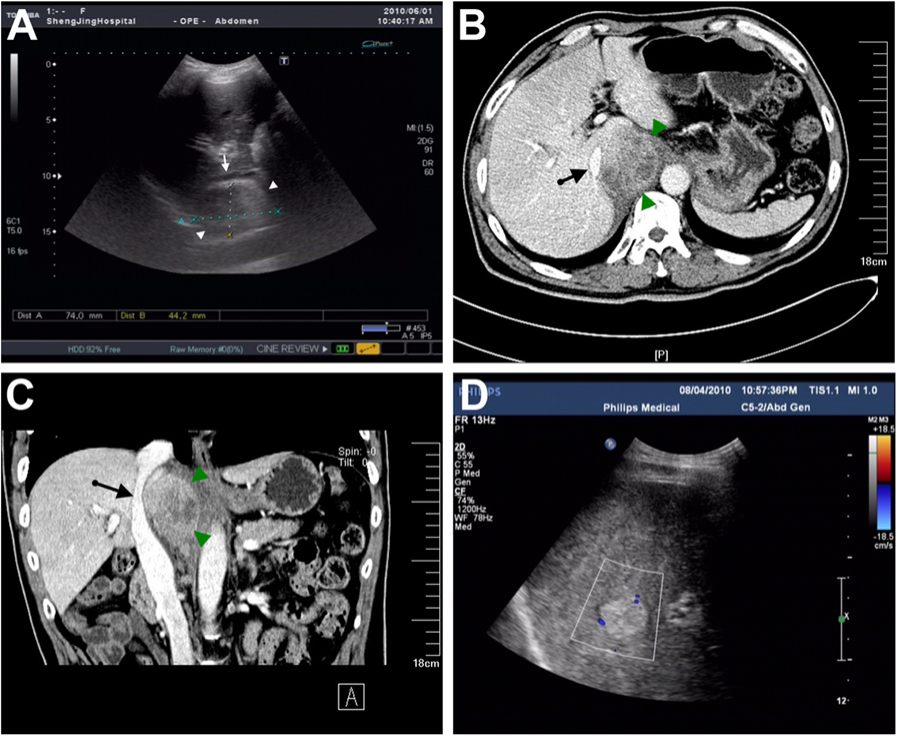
Diagnostic imaging of the retroperitoneal paraganglioma. (A) Abdominal ultrasonography showed a retrocaval mass (indicated by arrowheads) present in the right parasagittal sonographic section. The mass compressed the IVC (indicated by an white arrow) in an anterior direction. (B) Axial contrast-enhanced CT scan showed a non-homogeneously enhanced retrocaval mass (indicated by arrowheads) with necrotic cysts. (C) Coronal contrast-enhanced CT scan showed anterior displacement and extrinsic compression of the IVC (indicated by an black arrow) by the retrocaval mass (indicated by arrowheads). (D) Postoperative abdominal US scan showed multifocal hepatic metastatic lesions (shown in blue).
